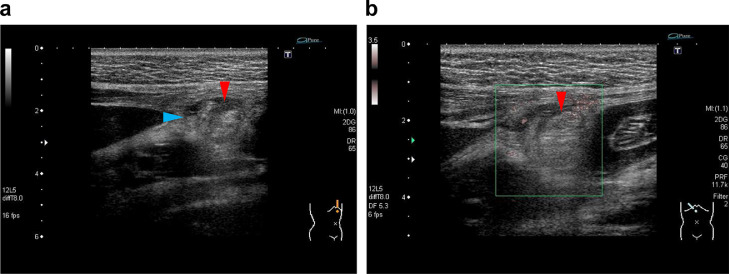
Ultrasound findings in Case 2. (A) There is a 17 × 15-mm oval-shaped hyperechoic mass adjacent to the falciform ligament with a peripherally located hypoechoic rim (red arrow head) and hyperechoic surrounding fat (blue arrow head). (b) No internal vascularity signal is seen on Doppler evaluation.
